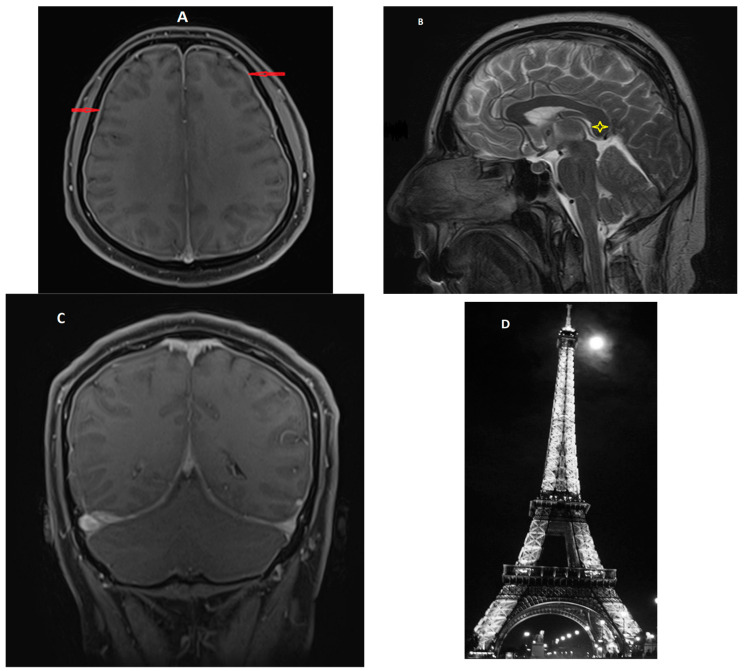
Initial brain MRI of the patient. (A) Axial contrast-enhanced T1-weighted image showing diffuse pachymeningeal enhancement (red arrows). (B) Sagittal T2-weighted image showing signs of brain sagging (slight downward displacement of the splenium of the corpus callosum, droopy penis sign—yellow star). (C) Coronal contrast-enhanced T1-weighted image showing enhancement of the pachymeninges (“Eiffel by night” sign) and engorgement of the sigmoid sinus. (D) Image of the Eiffel Tower at night.
